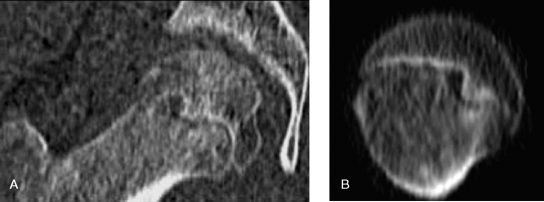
AP (A) and axial (B) CT scanograms of the right hip of the 13-year-old boy whose radiograph is shown in Figure 1A, now revealing an epiphyseal peg that seems to be a rather loose fit in the metaphyseal socket.
